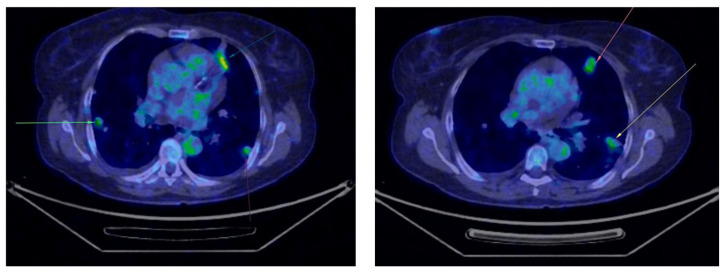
Second PET-CT scan showing the progression of the lung lesions (marked with arrows).
